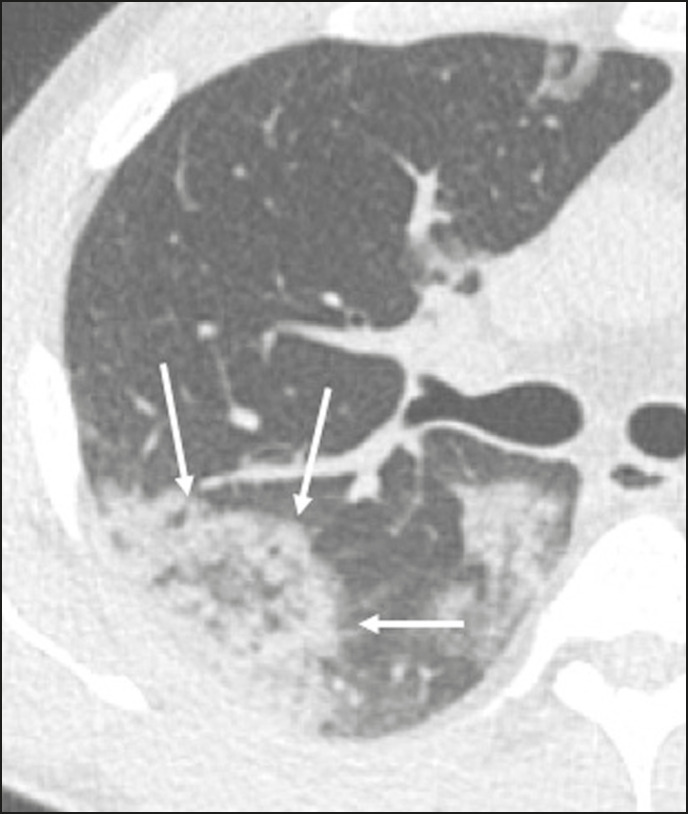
Chest CT scan of a 59-year-old male patient who was treated in the intensive care unit for 12 days with a diagnosis of COVID-19, showing pulmonary opacities, one with an inverted halo sign (arrows), characterized by consolidation with a low-attenuation center.
